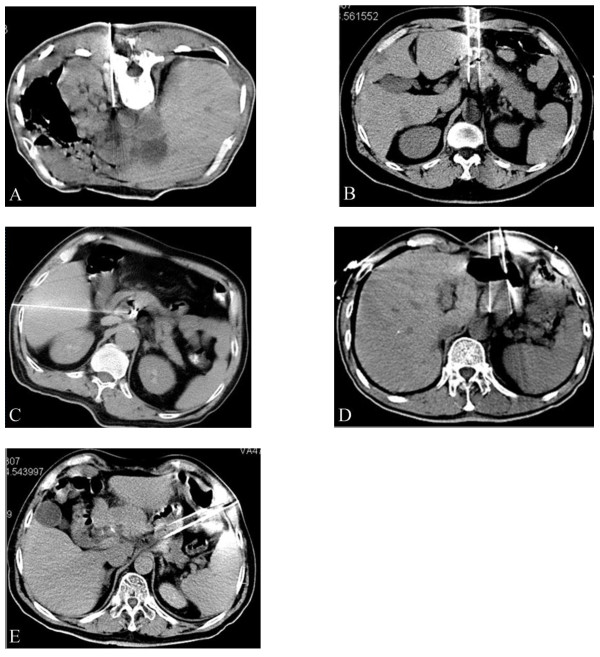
Iodine-125 seeds were implanted through percutaneous puncture under CT-guidance: a). Through the paraspinal space; b). Through the perirenal space between stomach and intestinal tract; c). By penetrating the liver; d). By traversing the stomach. e). By traversing the colon
